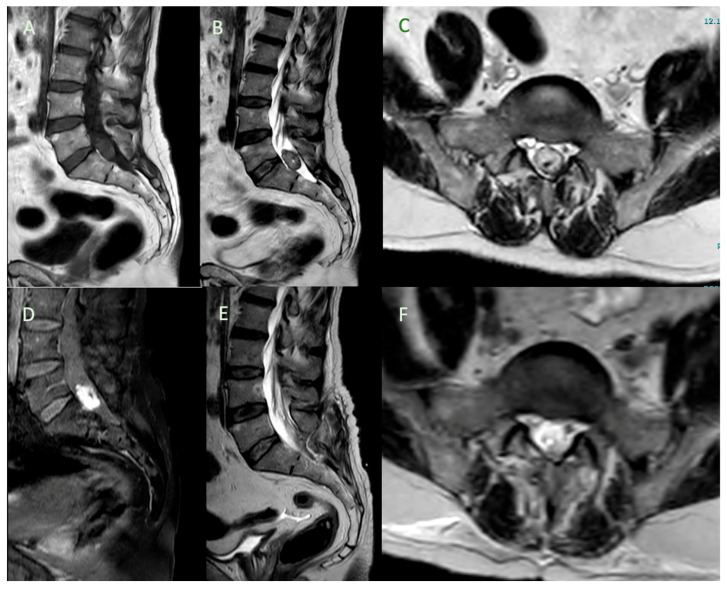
Preoperative and postoperative MRI findings of Case 4. Sagittal and axial preoperative T1-, T2-, and contrast-enhanced T1-weighted images (A–D) demonstrate an intradural extramedullary lesion at the S1 level with heterogeneous enhancement. Postoperative images (E,F) confirm gross total resection without residual lesion.
